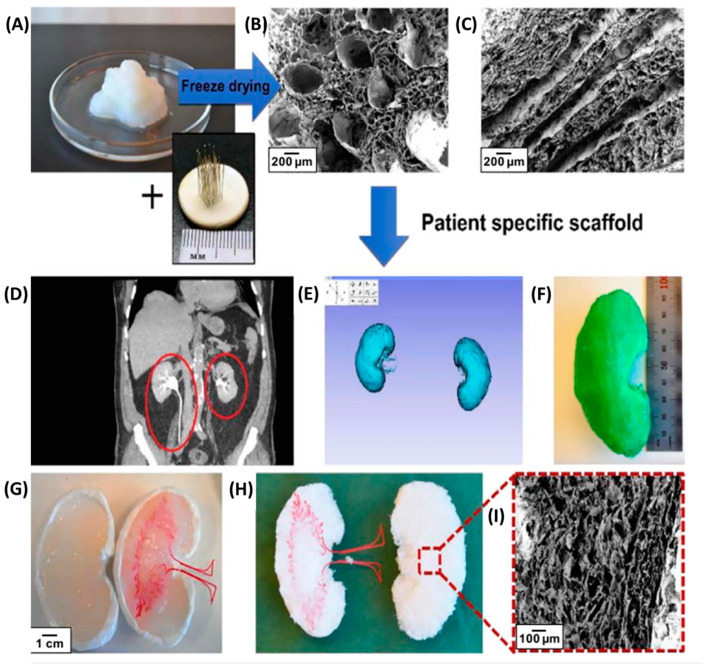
(A) BNC blended with alginate, (B) SEM image of homogenized BNC freeze-casted around clay-needle construct perpendicular cut to the channels, (C) cross-sectional cut of the channels, (D) abdominal computed tomography (CT) image with kidneys marked in red. The data have been anonymized, (E) STL-file of kidneys displayed in software Slicer, (F) printed PLA-kidney template, (G) clay-negative filled with homogenized BNC/alginate with an inserted PLA sacrificial template vascular tree, (H) freeze-dried BNC/alginate sponge cut in half holding a PLA sacrificial template vascular tree prior to the removal of the template by hydrolysis, (I) SEM image of sponge microstructure [217]. Copyright 2019 IOP Publishing Ltd.
